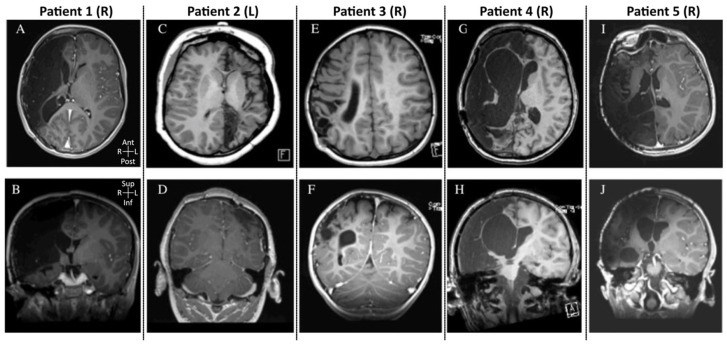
(A–J) Preoperative axial (top) and coronal (bottom) T1-weighted MRI images for patients 1–5, indicating the affected hemisphere (R: right, L: left). The figure itself labels each column as Patients 1–5, alongside the affected hemisphere labeled (and defined in the figure caption). Top and bottom images are defined as axial and coronal per the figure caption.
